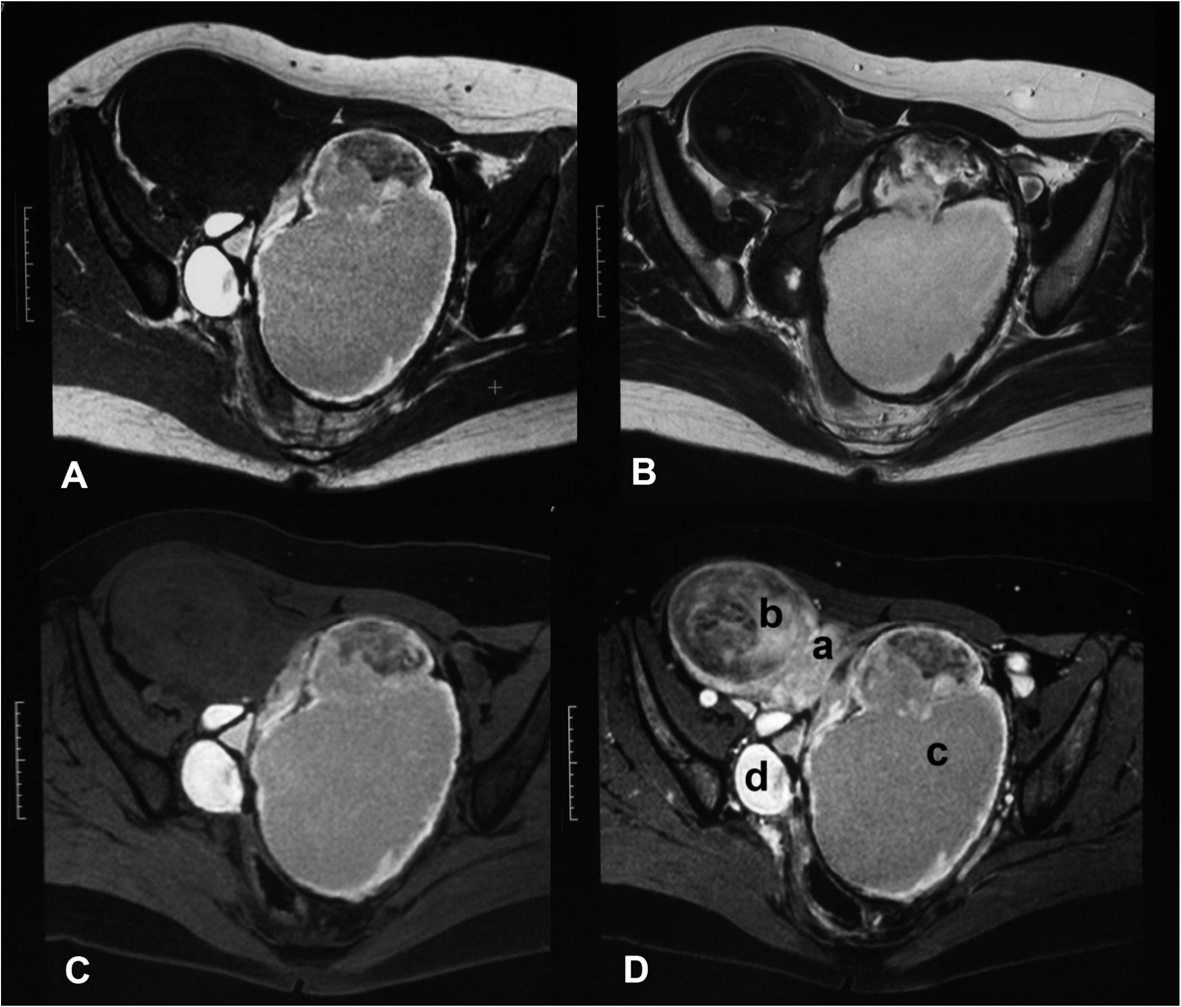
Pelvic magnetic resonance images of our patient before radiotherapy. (A) T1-weighted image; (B) T2-weighted image; (C) fat-saturated T1-weighted image; (D) fat-saturated contrast-enhanced T1-weighted image. (a) Normal uterine body; (b) subserous myoma uteri; (c) huge chocolate cysts (endometrial cysts) arising from the left ovary; (d) endometrial cysts arising from the right ovary.
